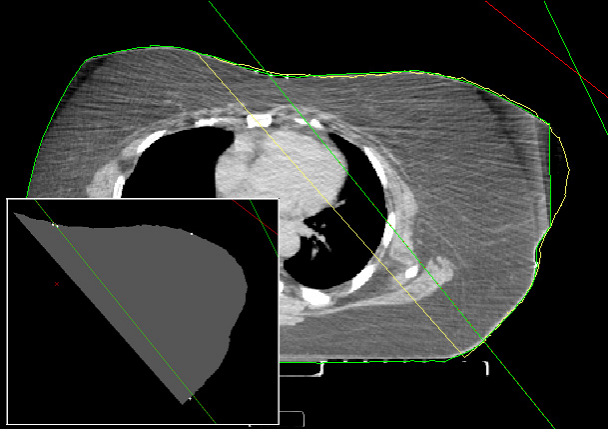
A case example of a left‐breast treatment where CT‐generated body contours (green) did not include the entire breast. The dose calculation had to be carried out using the body contours obtained from the 3D digitization system (yellow).
